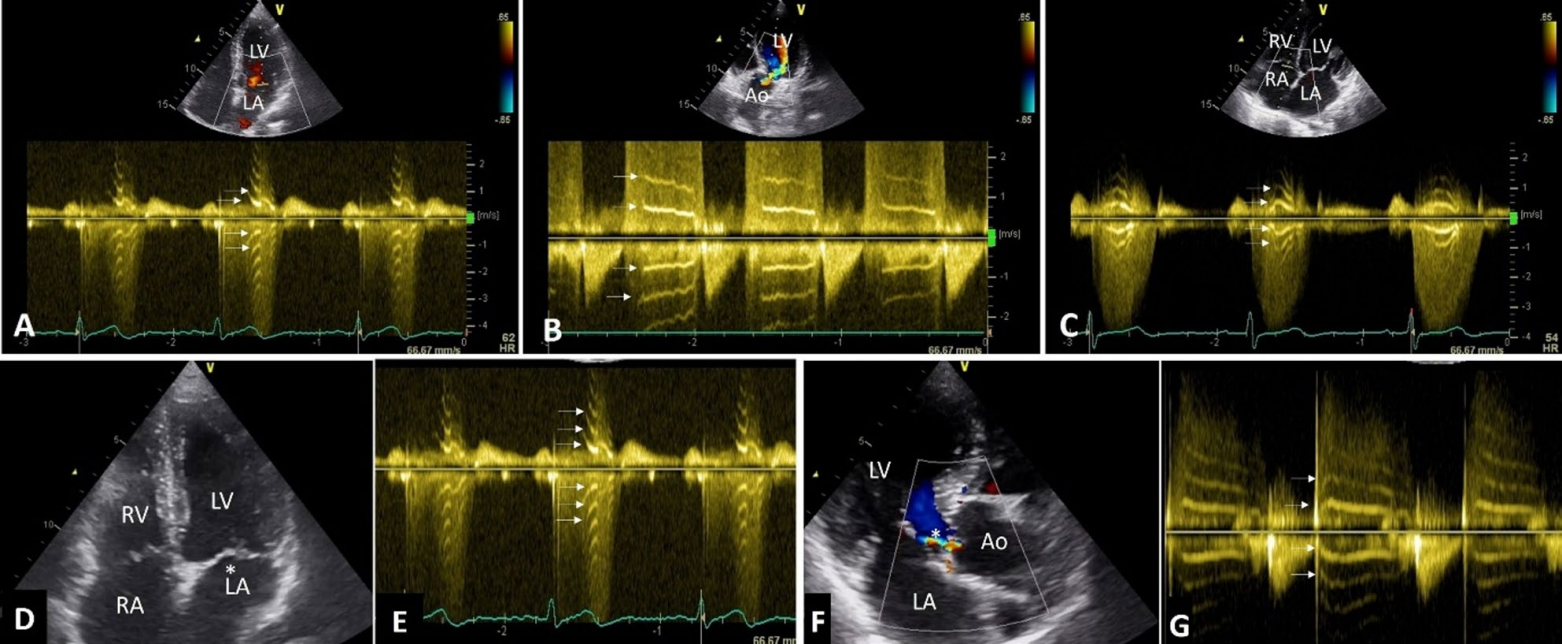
Transthoracic echo showing tiger stripes (horizontal arrows) in left sided regurgitant lesions in (A) apical 4 chamber view showing a CW spectrum of mitral regurgitation, (B) Apical 5 chamber view showing CW Doppler spectrum of aortic regurgitation. (C) Apical 4 chamber showing high velocity tricuspid regurgitant jet due to pulmonary hypertension. (D-G). Tiger stripes without oscillating structure in regurgitant pathway. (D) Apical 4 chamber view does not show any oscillating mass (*) in a case of mild mitral regurgitation (see video 1 and video 2). (E) CW Doppler spectrum of the same case shows tiger stripes (arrows) (F) No oscillating mass is seen in aortic regurgitation pathway (*), (G) Same case showing tiger stripes in mild aortic regurgitation spectrum (arrows), (see video 3). RV-Right ventricle, LV-Left ventricle, RA-Right atrium, LA-Left atrium, Ao-Aorta
